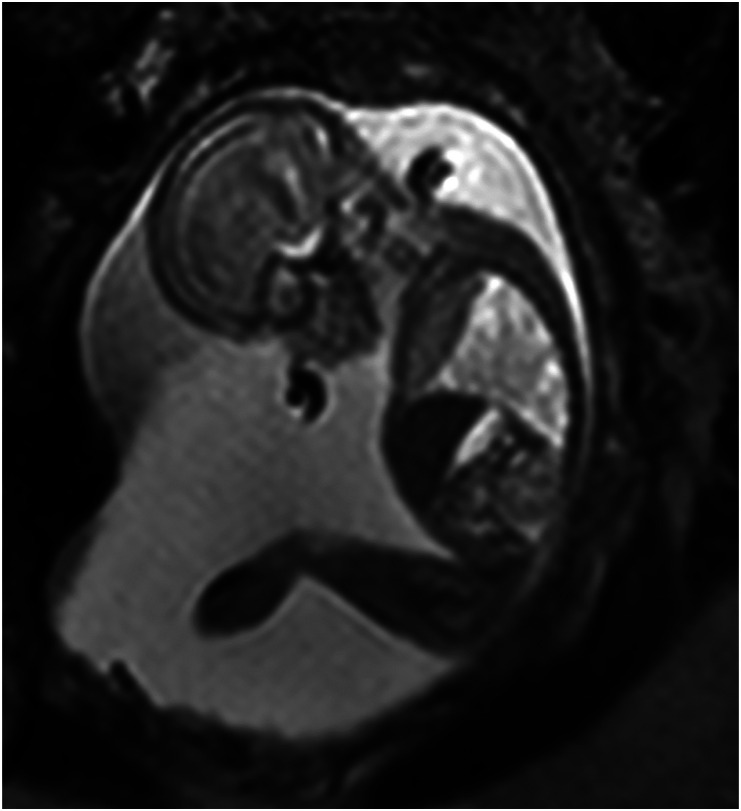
Case no 174. At ultrasound examination, there was a suspicion of left-sided diaphragmatic hernia. Sagittal MR image shows intact diaphragm, but signal intensity changes suggesting congenital cystic adenomatoid malformation in the inferior left lung lobe.
